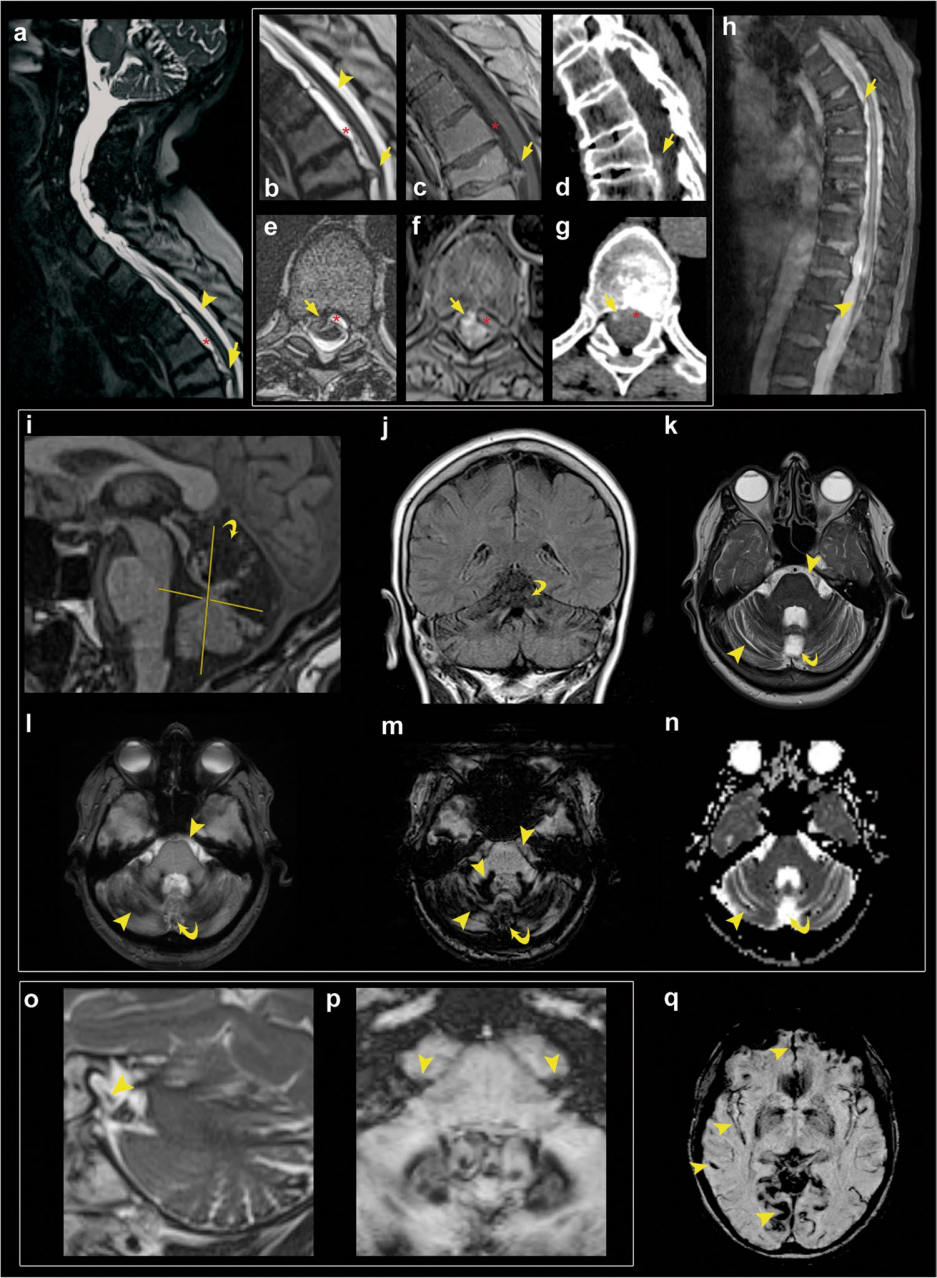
Non-contrast sagittal Constructive Interference in Steady State (CISS) (a), Turbo-Spin Echo (TSE) T2 (b), and T1 (c) Magnetic Resonance (MR) images of the cervical and upper thoracic spine show an anterior wedge degenerative deformation of the D6 vertebral body with superimposed a prominent central disc extrusion characterized by osteo-calcific signal (yellow arrow) determining a dural tear at D6-D7 level. Above the disc extrusion, note the “sentinel” epidural fluid collection (red asterisk) stretching along the ventral aspect of the spinal canal and displacing the dura posteriorly. The partially calcified disc extrusion is also recognizable by non-contrast sagittal spinal Computed Tomography (CT) images (d). Axial T2 (e) and T1 (f) TSE as well as CT (g) images through the D6–D7 disc clearly show a dural defect just on the right of the midline at the level of the spur and further delineate the associated ventral epidural fluid collection. CISS (a) and TSE T2 (b, e) MR images show low signal intensity along the surface of the spinal cord consistent with superficial siderosis (SS, yellow arrowhead); extensive SS around the spinal cord, also below the dural defect and up to the medullary cone, is best demonstrated by Sagittal Gradient-Echo (GRE) T2 MR images of the thoracic spine (h). Non-contrast sagittal 3D T1 (i), coronal T2 Fluid-attenuated inversion recovery (FLAIR) (j), axial TSE T2 (k), GRE (l), susceptibility weighted (SW) (m), and apparent diffusion coefficient (ADC) (n) images show a specific pattern of superior cerebellar atrophy (yellow crooked arrow) associated with infratentorial diffuse SS (yellow arrowhead), mostly of the pons, cerebellar folia, dentate hila, and superior vermis. SS along the eighth cranial nerves on both sides is clearly seen by Sagittal TSE T2 (o) and axial GRE (p) magnification. Supratentorial SS (yellow arrowhead) along the interhemispheric and Sylvian fissures, as well as within temporal and occipital sulci is well demonstrated by the axial susceptibility weighted (SW) sequence (q)
